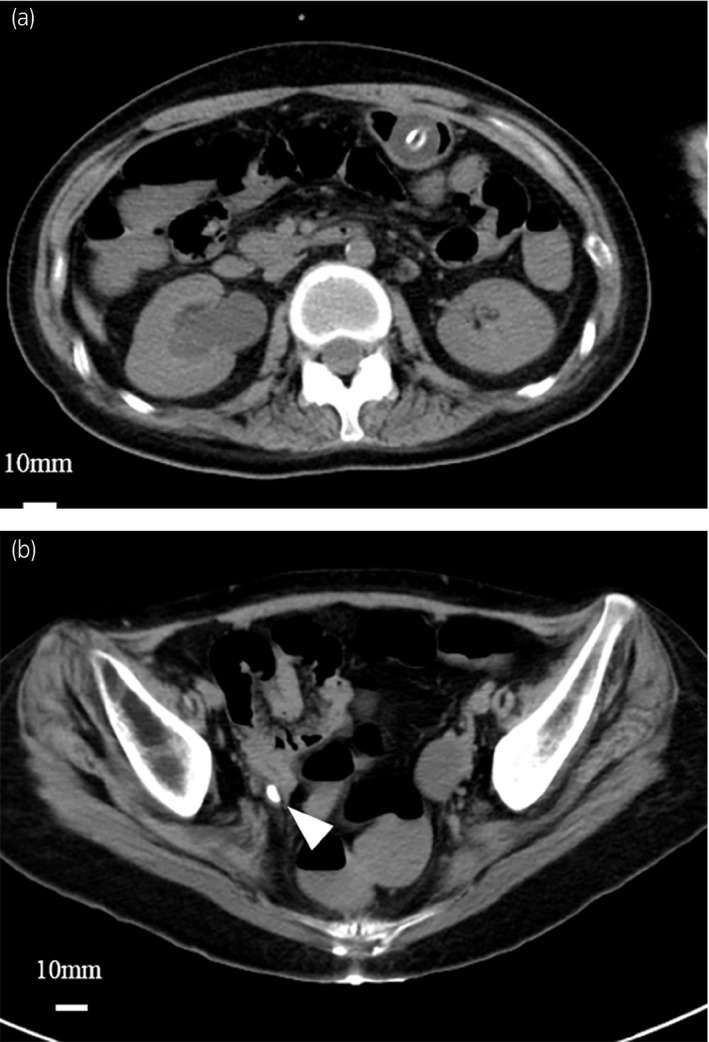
Abdominal unenhanced computed tomography demonstrates (a) hydronephrosis and (b) ureteral stone in the right distal ureter (white arrows).
